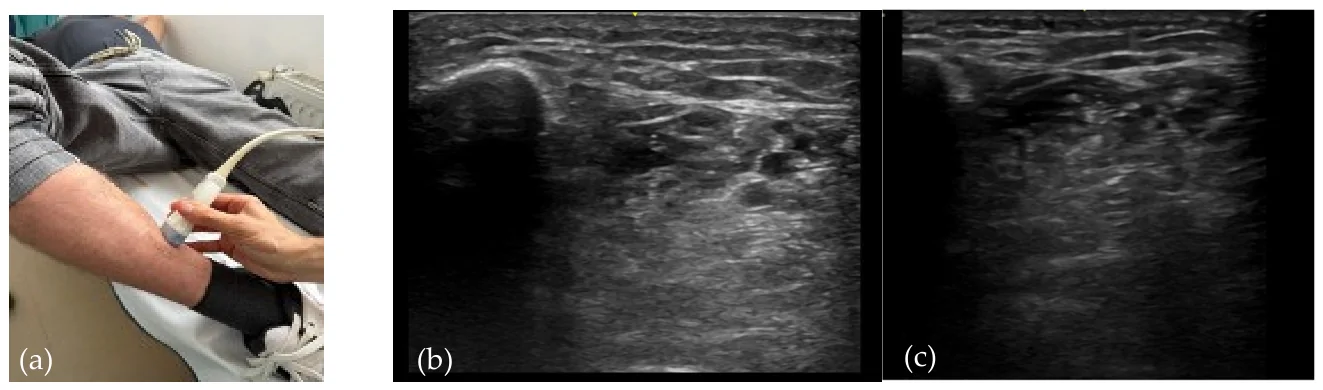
MSK US probe placement. Tibialis posterior muscle. (a) Patient positioning and probe placement at the distal third of the calf in cross-sectional plan. (b) MSK US image of tibialis posterior muscle at T0. Baseline ultrasound image demonstrating the tibialis posterior (TP), flexor hallucis longus (FHL), and flexor digitorum longus (FDL) muscles. Due to chronic post-stroke muscular changes, differentiation of individual muscle boundaries may be challenging, highlighting the importance of dynamic and ultrasound-guided assessment. (c) MSK US image of tibialis posterior muscle at T1. Follow-up ultrasound image obtained at 3 months using the same acquisition protocol. Persistent increases in echogenicity and architectural alterations remained visible, although slight improvement in muscle echotexture was observed. Overall structural changes remained relatively stable compared with baseline. These muscles were selected for multimodal intervention based on the combined interpretation of clinical findings, pain presentation, functional limitations, and ultrasound characteristics. Abbreviations: MSK US: Musculoskeletal ultrasound; T0: baseline assessment; T1: follow-up at 3 months.
